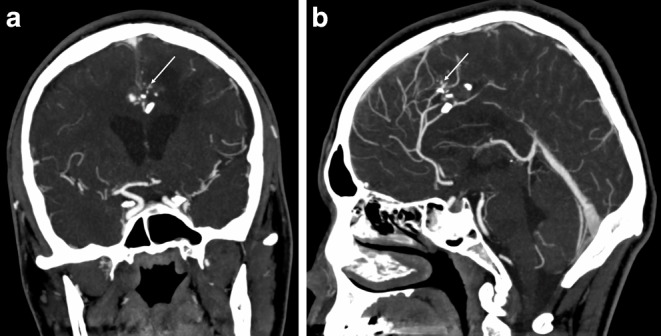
CT angiography of the cerebral arteries in coronal (a) and sagittal (b) views with traumatic aneurysms and bone remnants in the projection of the pericallosal arteries (*arrows*)
